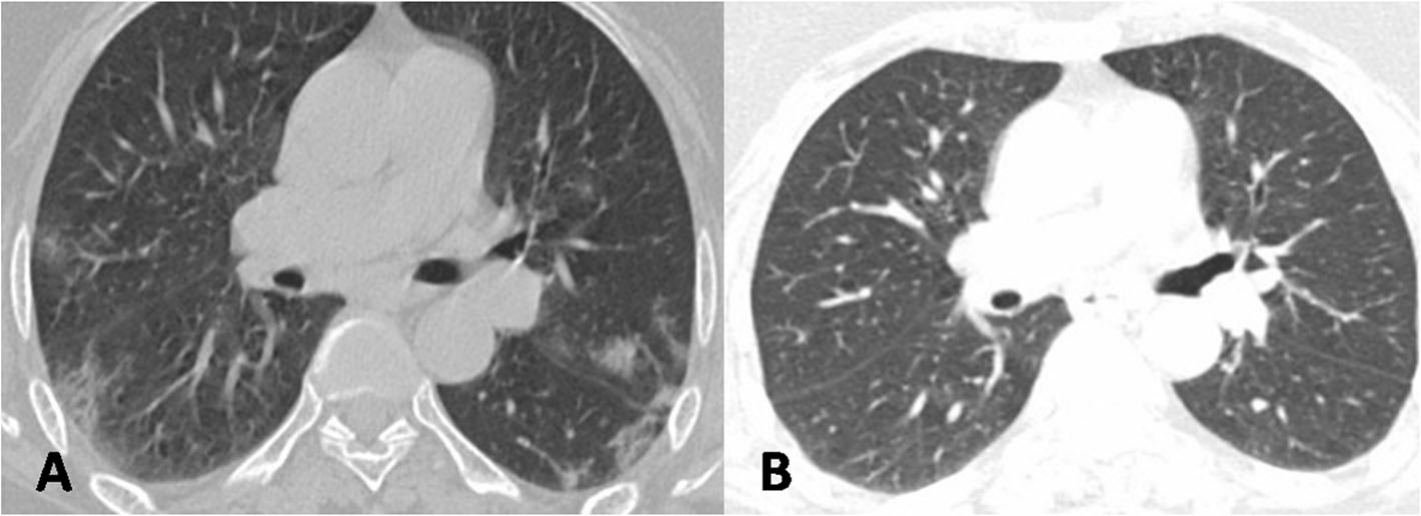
Axial chest CT image in lung window settings of a 49-year-old COVID-19 patient showing multifocal subpleural ground glass opacities (GGOs) in both lungs at the time of hospital admission (a). Follow-up CT performed 93 days after the first CT reveals complete resolution of GGOs (b)
